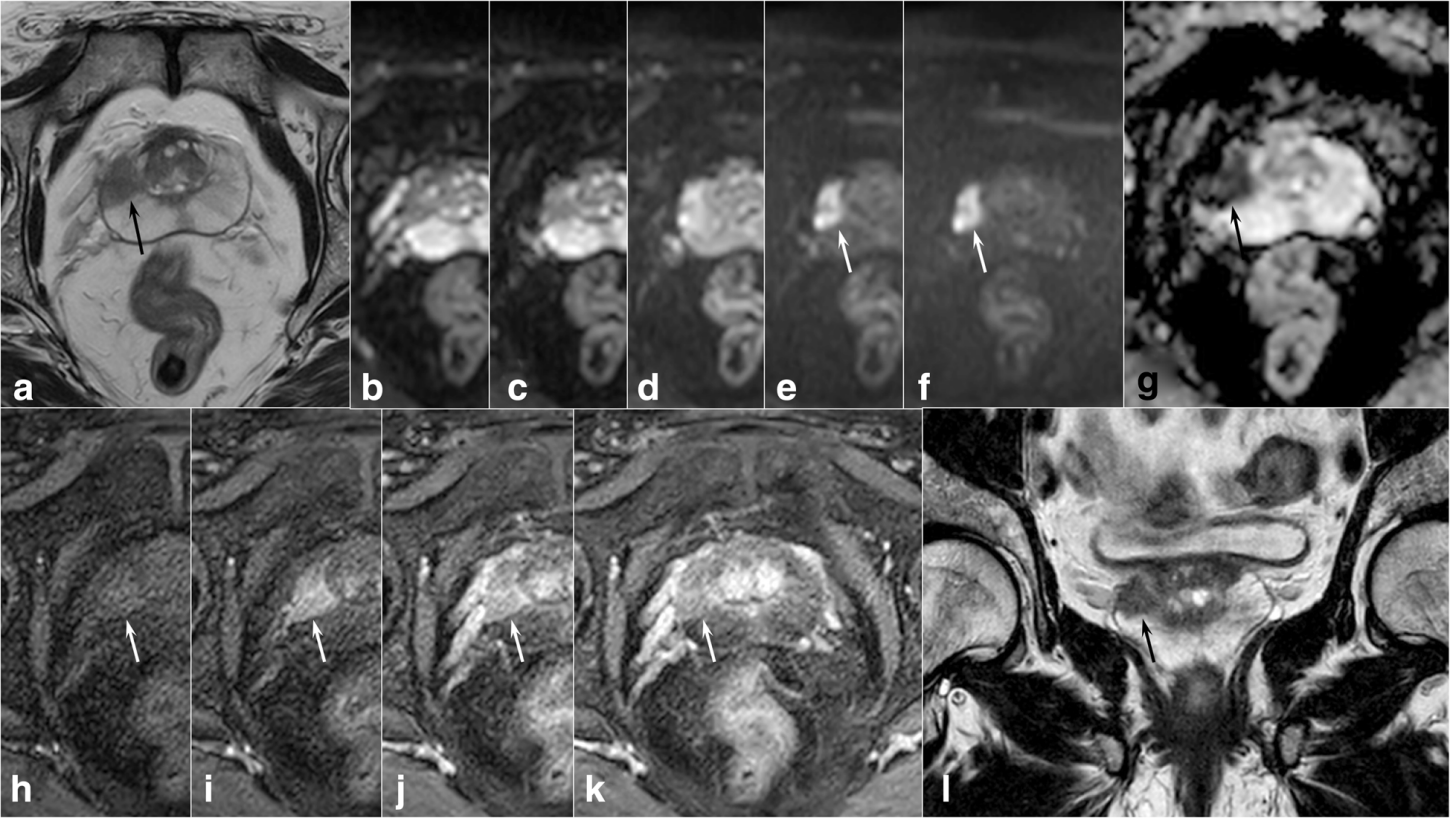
Prostate multiparametric magnetic resonance imaging showing a neoplastic lesion of the right peripheral zone (arrows). The lesion is hypointense on axial (a) and coronal (l) T2-weighted images and demonstrates diffusion restriction on diffusion-weighted images (*b* values 0, 100, 500, 1000, and 1400 s/mm^2^, from b to f, respectively), confirmed by the apparent diffusion coefficient map (g). Lesion enhancement is also evident on dynamic contrast-enhanced perfusion-weighted imaging (from h to k). PI-RADSv2 diagnostic category: 5
